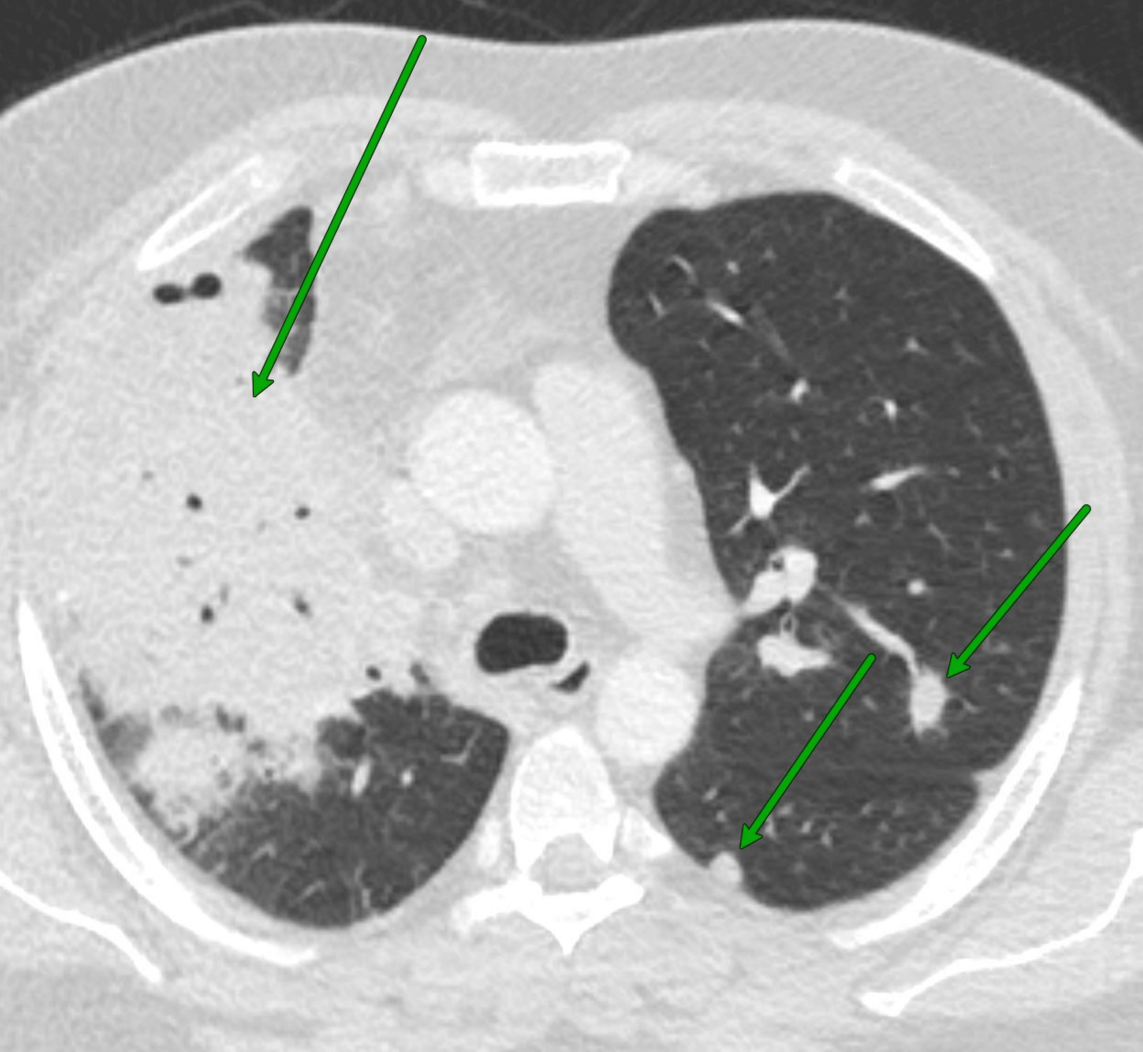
Chest CT of Case 2 Dominant right upper lobe consolidation, as well as infectious-appearing nodules in both the left upper and lower lobes marked by green arrows CT: computed tomography
